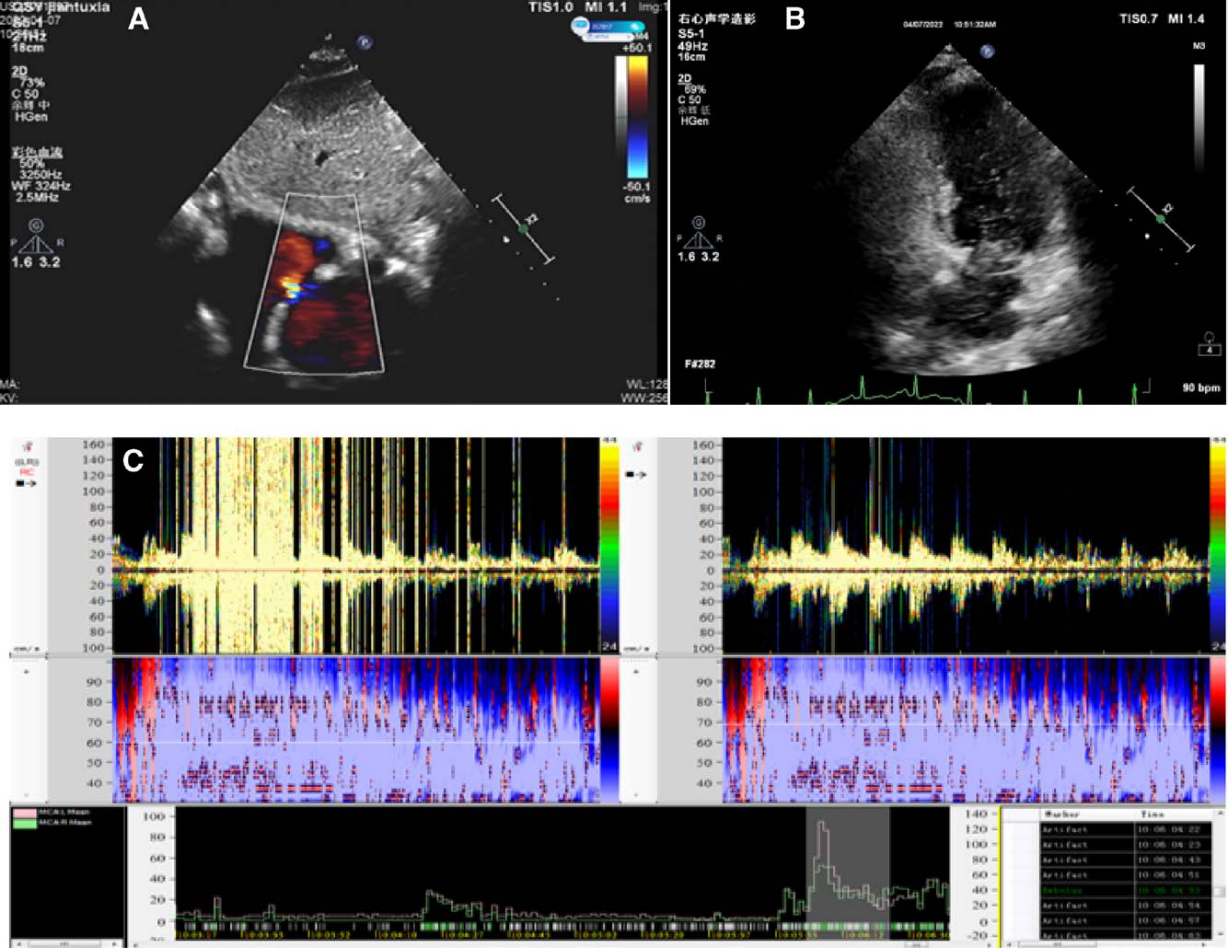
(A–B) TTE showed echo separation at the oval of the atrial septum. The atrial was horizontal and detectable, and left-to-right shunt signal was observed. TTE right echocardiography showed a small amount of contrast agent reflection in the left atrium and left ventricle in the resting state. A large amount of contrast agent reflection was found in the left atrium and left ventricle in the 3 cardiac cycles after Valsalva. TCD foaming test (C): The double-depth blood flow signal of the left vertebral artery was monitored (60–69). The medium embolus signal was detected by pushing the activated saline under the resting state, and rain curtain embolus signal was detected within 12 seconds after Valsalva operation. TCD = trancranial doppler, TTE = transthoracic echocardiogram.
